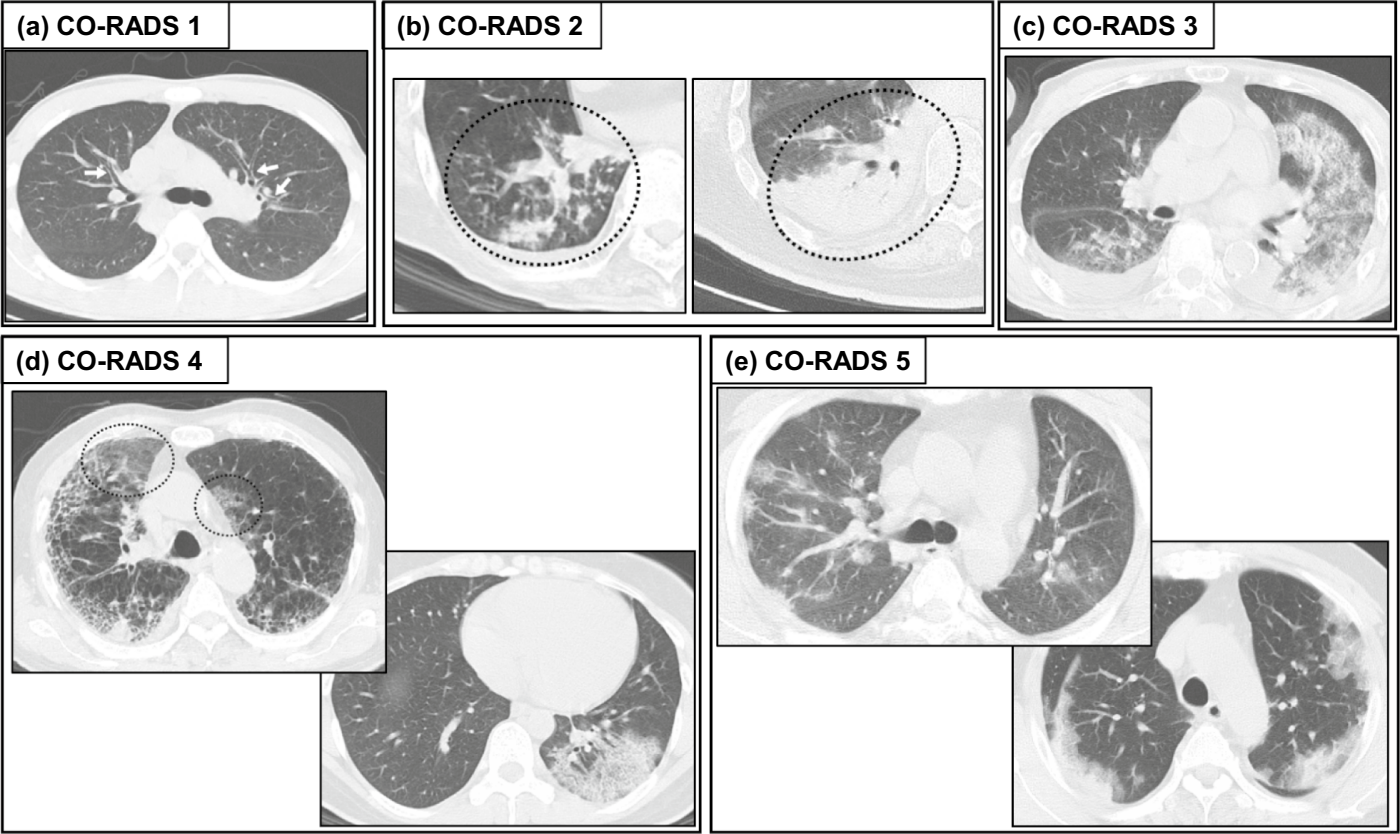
Example of chest CT patterns of CO-RADS. Axial CT images are categorized as (a) CO-RADS 1, with no features of pneumonia, (b) CO-RADS 2, with features typical for infection other than COVID-19, e.g., bronchial pneumonia, lobar pneumonia, tuberculosis, or fungal infection, (c) CO-RADS 3, with features compatible with COVID-19 but also other diseases, e.g., alveolar pulmonary edema, (d) CO-RADS 4, with features suspicious for COVID-19 but with overlap with other diseases, drug-induced pneumonia or collagen disease-related lung diseases, and (e) CO-RADS 5, with typical pattern of COVID-19 pneumonia [49]
